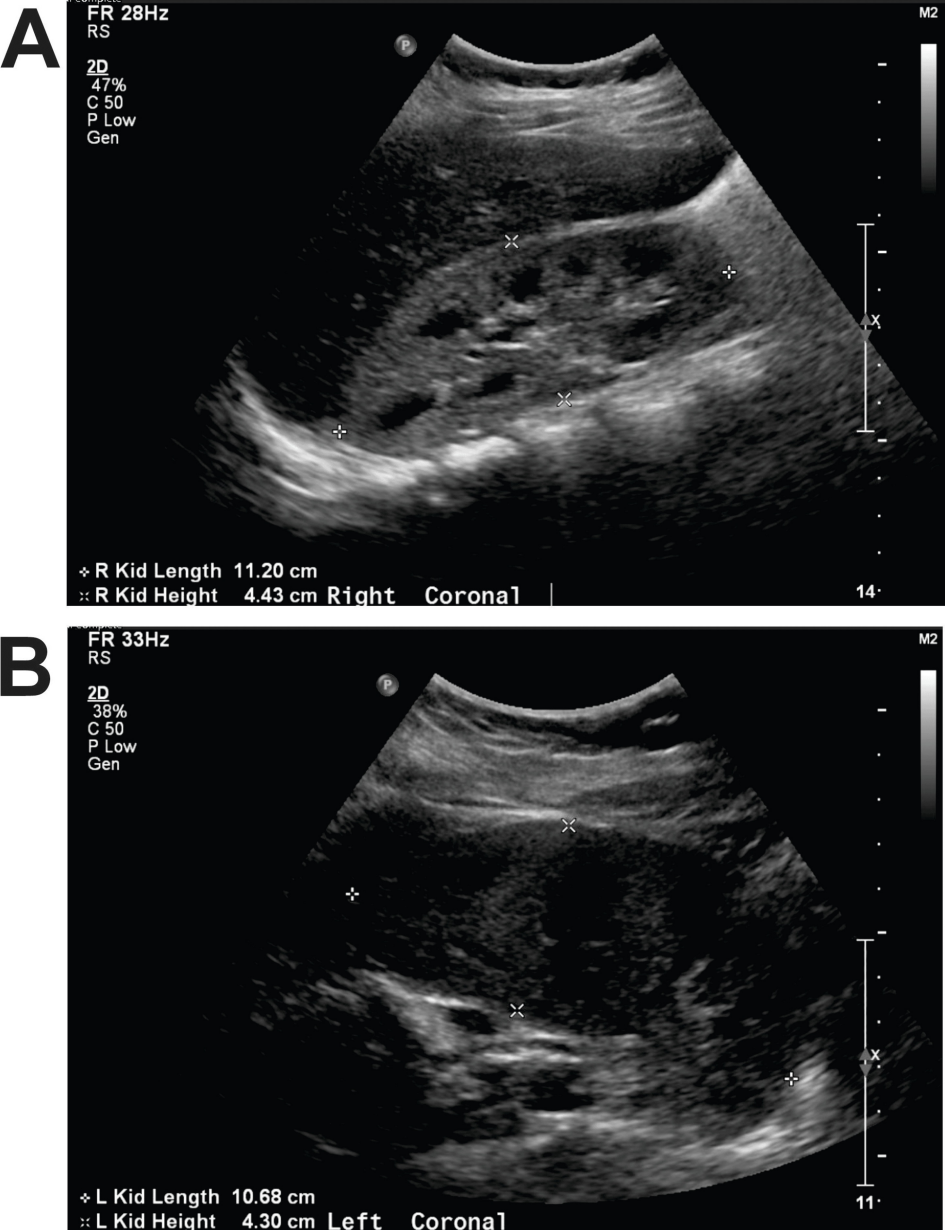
Renal ultrasound at admission. Transverse views of the right (A) and left (B) kidneys demonstrate bilaterally enlarged kidneys with diffuse parenchymal hyperechogenicity, consistent with medical renal disease. No hydronephrosis is seen.
